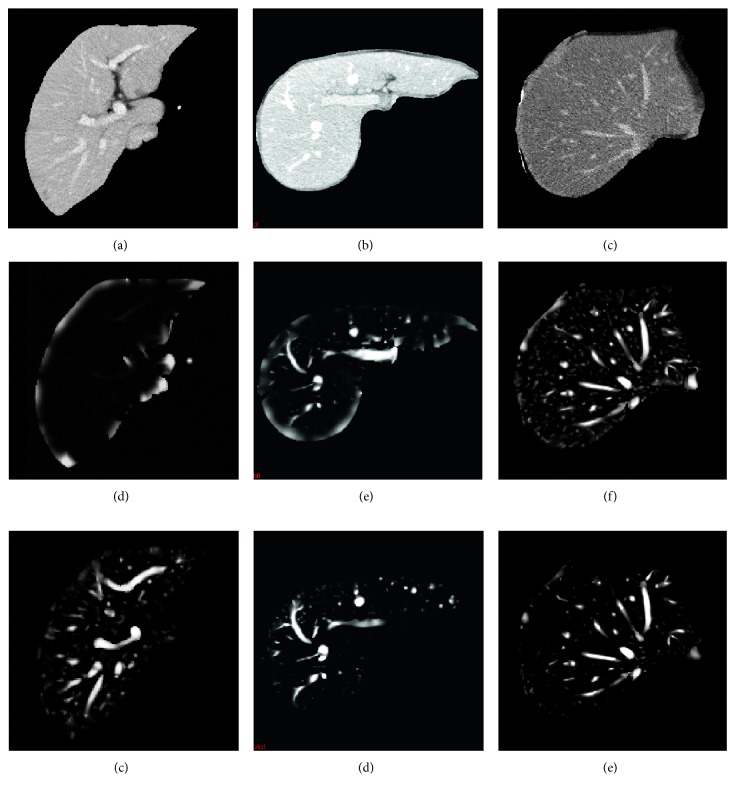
Comparison of the improved vesselness filter with the Jerman's vesselness filter. (a)–(c) The original CT images. (d)–(f) The vesselness images by using the Jerman's vesselness filtering to (a)–(c), respectively. (g)–(i) The vesselness images by using the improved vesselness filtering to (a)–(c), respectively.
